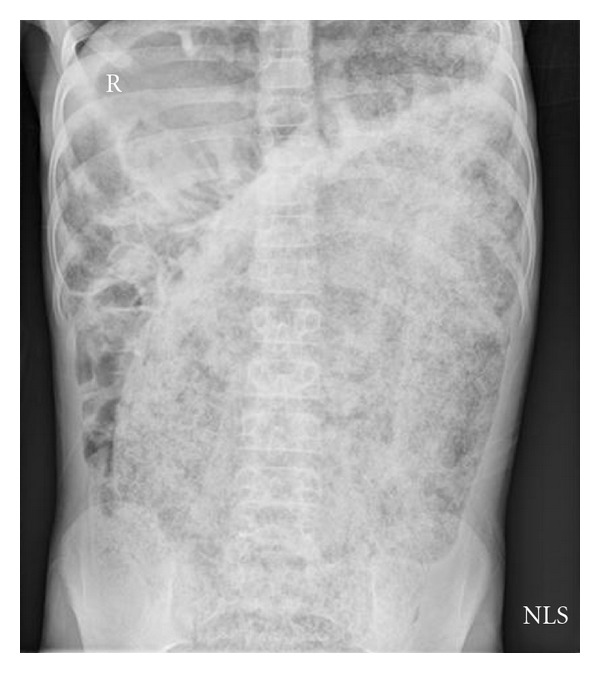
Abdominal X-ray at first presentation confirming profound constipation with large fecaloma.
